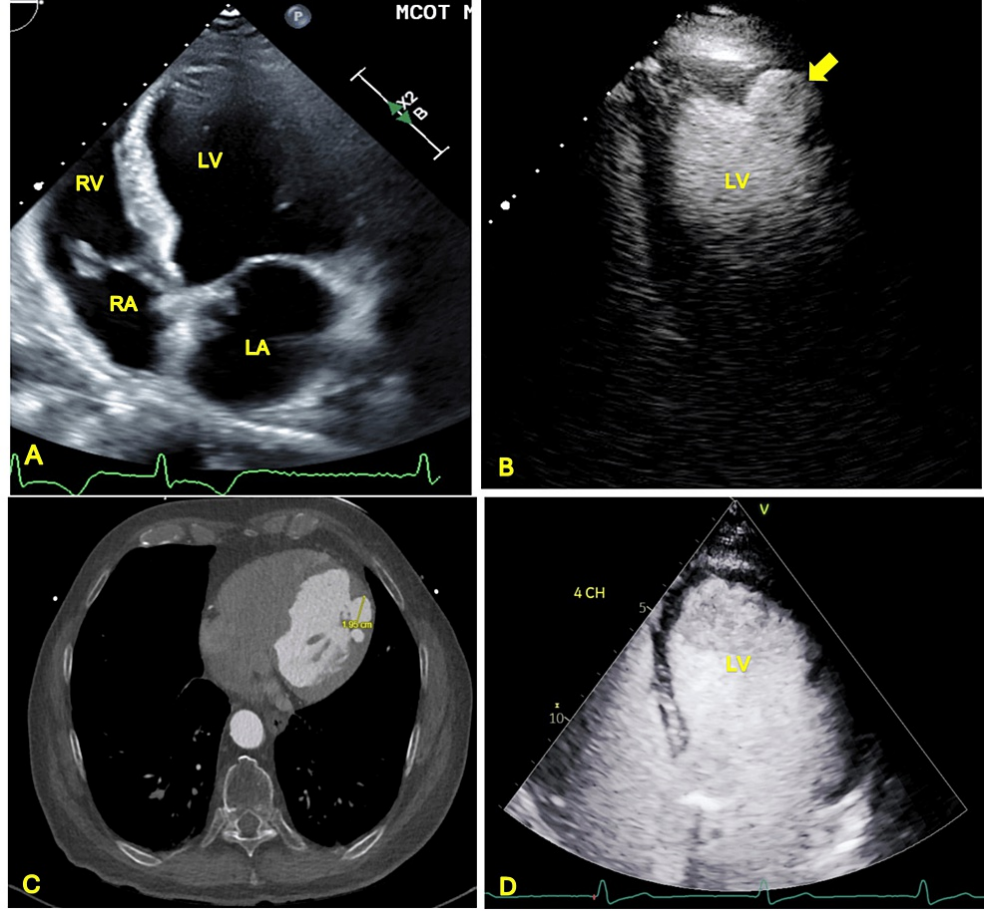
(A) Apical four-chamber view of transthoracic echocardiogram without contrast enhancement. Pseudoaneurysm was not apparent in this view. (B) Apical four-chamber view with contrast enhancement demonstrating left ventricular pseudoaneurysm (yellow arrow) in the distal anterolateral wall. (C) Contrast-enhanced CT demonstrating pseudoaneurysm in the distal anterolateral wall of the left ventricle measuring 1.95 cm at its largest diameter. (D) Apical four-chamber view of transthoracic echocardiogram with contrast enhancement post pseudoaneurysm repair. This repeat image demonstrates that pseudoaneurysm has resolved with surgical repair. LV: left ventricle, LA: left atrium, RV: right ventricle, RA: right atrium
